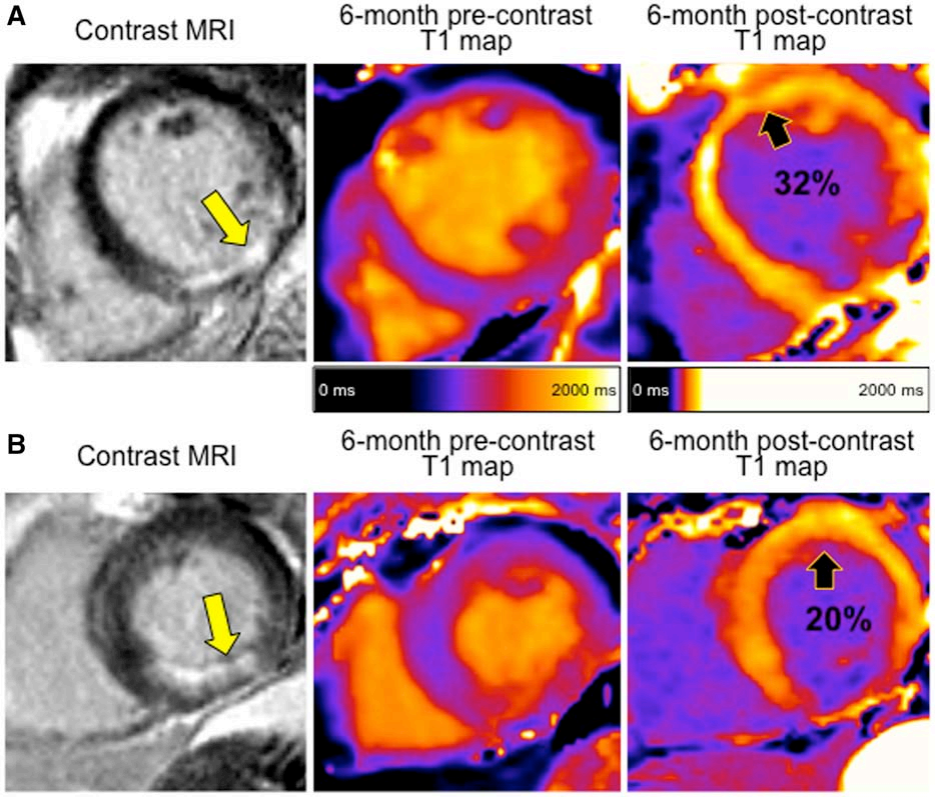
Two patients with similar presentations of acute ST-elevation myocardial infarction (STEMI). Both patients were treated by primary percutaneous coronary intervention and with the same medication. At the end of the procedure, both patients had thrombolysis in myocardial infarction coronary flow grade 3 in the culprit artery. A, Increasing remote zone extracellular volume (ECV). Cardiac magnetic resonance (CMR) performed 2 days post STEMI revealed a remote zone ECV of 28%. Remote zone ECV increased by 4% by 6 months to 32%. Left ventricular (LV) end-diastolic volume increased from 116 to 135 mL as measured by the 2-day and 6-month CMR scans. B, Decreasing remote zone ECV: CMR performed 2 days post STEMI revealed a remote zone ECV of 22%. Remote zone ECV decreased by 2% by 6 months to 20%. LV end-diastolic volume decreased from 128 to 102 mL as measured by the 2-day and 6-month CMR scans. MRI indicates magnetic resonance imaging.
